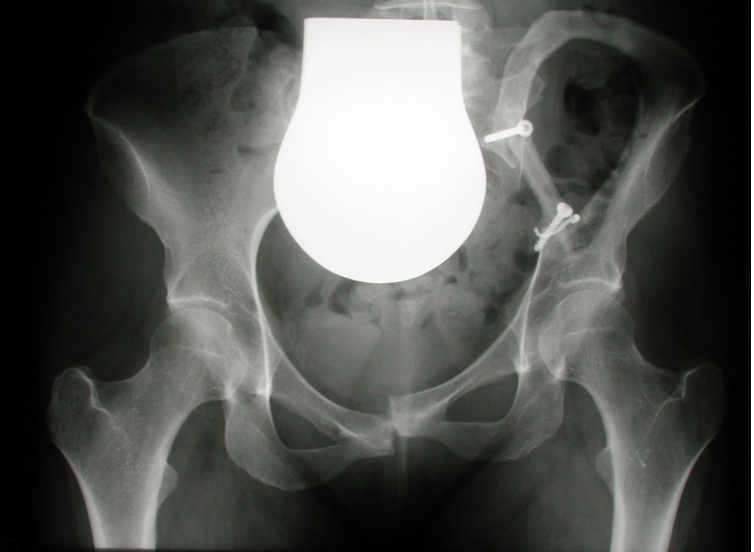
Radiographic check 18 months after surgery, showing identical position of the fibular transplant
